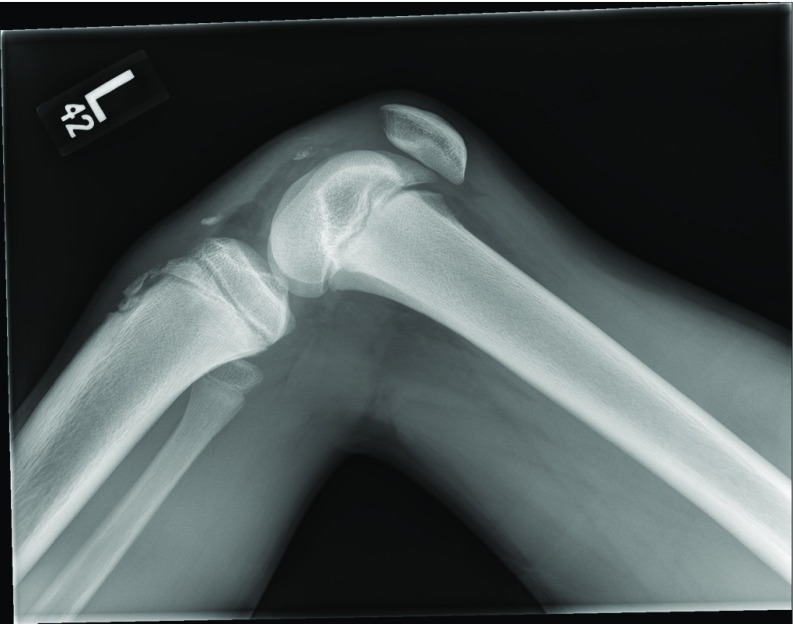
Lateral preoperative radiographs of the patient described in case 2. There is evidence of a displaced sleeve fracture from distal patella and a simultaneous avulsion from the tibial tuberosity accompanied by small bony fragments.
